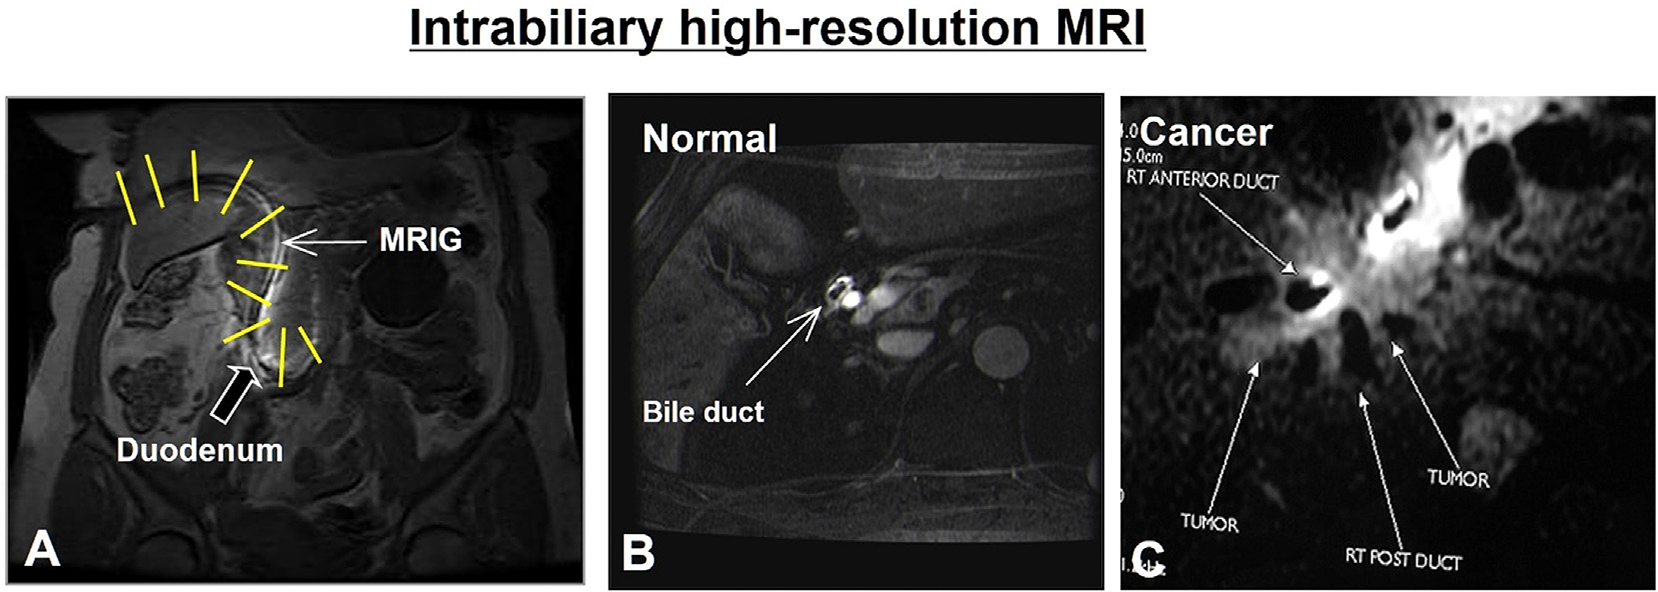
Intrabiliary MRI. (A) Coronal MRI showing MRIG (arrow) entry into the right bile duct, common bile duct, and duodenum through a percutaneous biliary drain, with intrabiliary MRIs captured along the MRIG (yellow lines). (B) Intrabiliary cross-sectional MRI of a normal bile duct as a “clean bright ring” (arrow B), compared to a bile duct with tumor infiltration (C) (Courtesy of Aravind Arepally).
